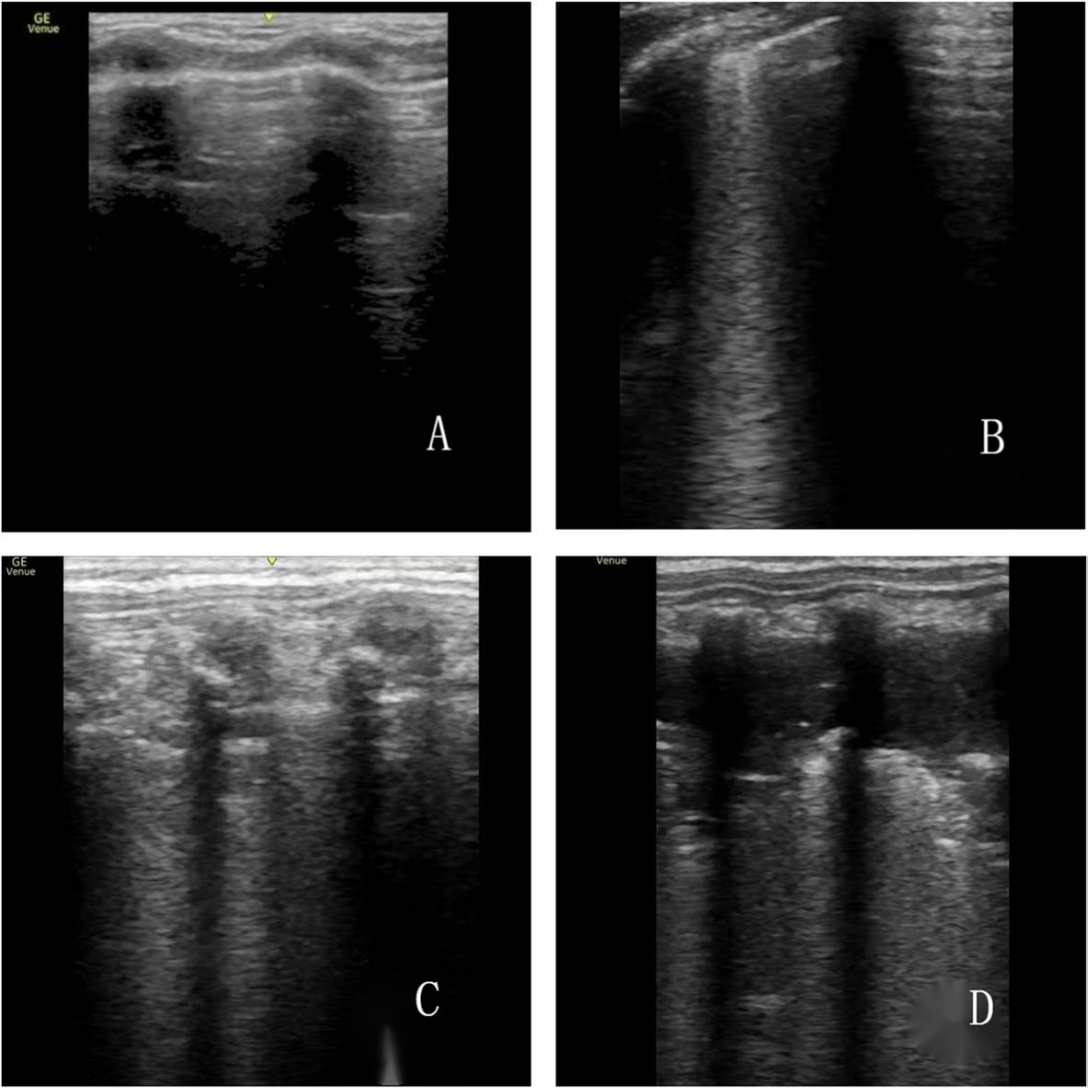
Ultrasound images under different lung ultrasound scores. A is 0 score ultrasound image, indicating normal signs; B is 1 score ultrasound image, indicating a small amount of B line; C is 2 scores ultrasound image, indicating diffuse fusion of line B; D is 3 scores ultrasound image, indicating extensive lung consolidation
